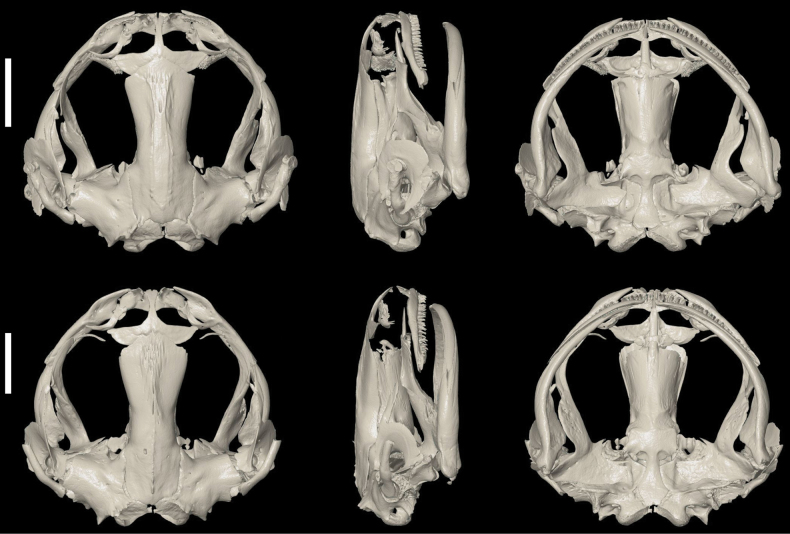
X-ray computed tomogram of the skull of adult *Xenopuslaevis* individuals from Comines-Warneton, Belgium in dorsal view, right profile and ventral view. Top = RBINS 18733 (male), bottom = RBINS 18736 (female). Scale bars: 5 mm. Scan by JB.
